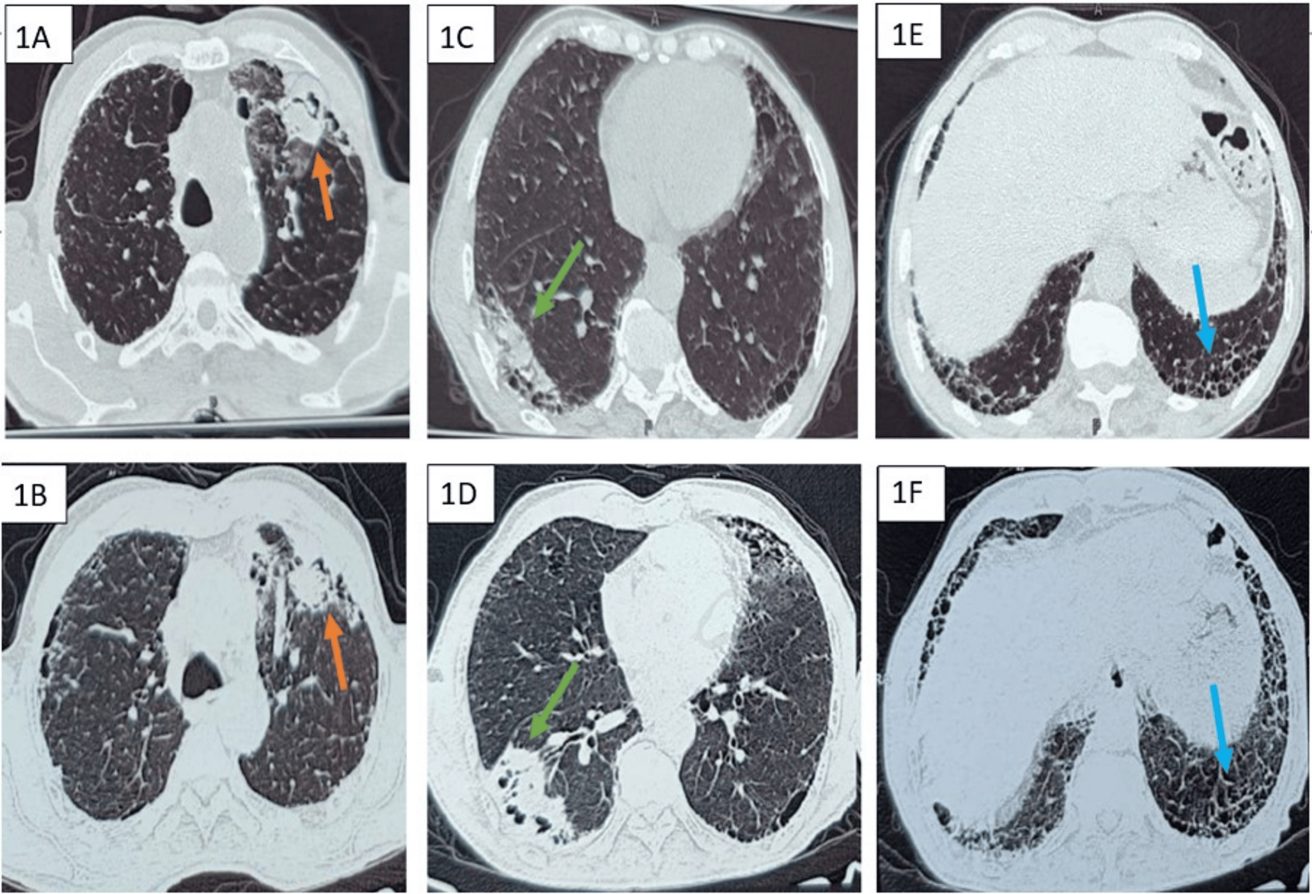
High-resolution computed tomography images of the patient The upper panel displays initial findings, and the lower panel shows follow-up images after three months. 1A & 1B: Cross sections at the level of the lower trachea show a left upper lobe mass-like lesion with an air crescent sign (arrow) and progressive increase in fibrosis. 1C-1D: Cross-sections at the level of cardiac chambers suggestive of a right lower lobe mass-like lesion (arrow) with progressive increase in size and subpleural fibrosis. 1E & 1F: Cross-sections at basal levels show bilateral subpleural septal thickening and honeycombing, suggestive of a definite usual interstitial pneumonia pattern (arrow).
